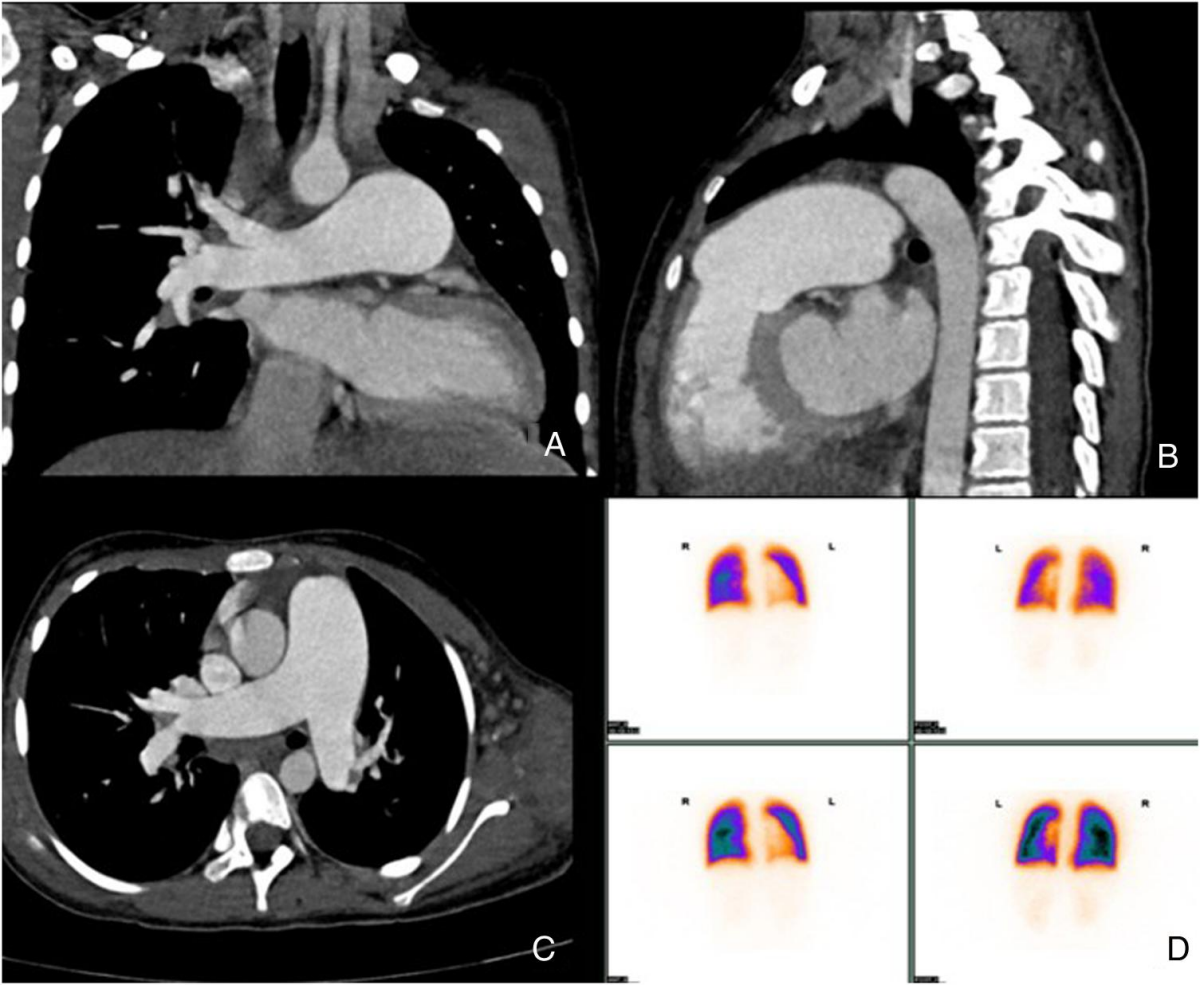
(A–C) Pulmonary artery enhanced CT shows total pulmonary artery dilation; (D) shows pulmonary perfusion imaging, demonstrating normal ratio of the left and right lung blood flow.
